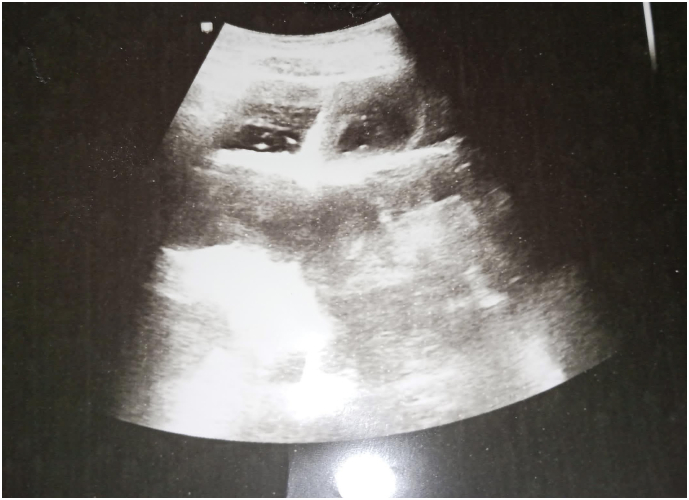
Abdominal ultrasound shows dilated small bowel with retrograde movements and a collapsed segment in the right iliac region. The liver, gallbladder, biliary tract, pancreas, spleen, and kidneys appear normal.
